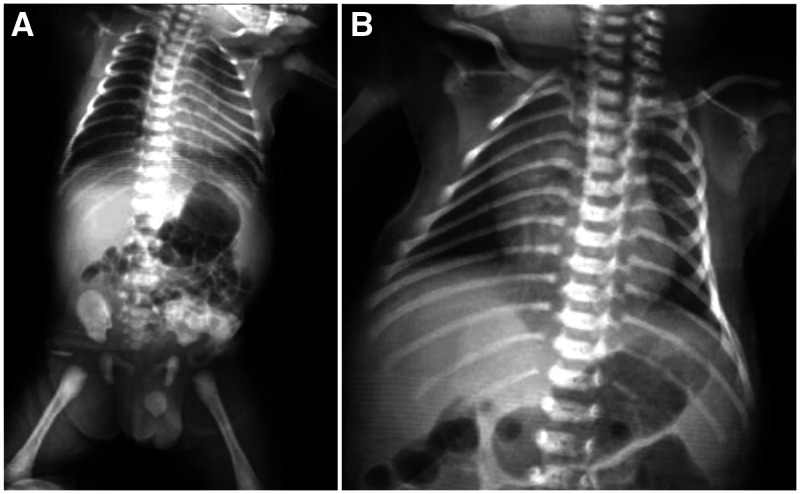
(A) Chest X-ray on day 1 of hospitalization is normal. (B) On day 6 of hospitalization, the chest X-ray indicates right pneumonia.
